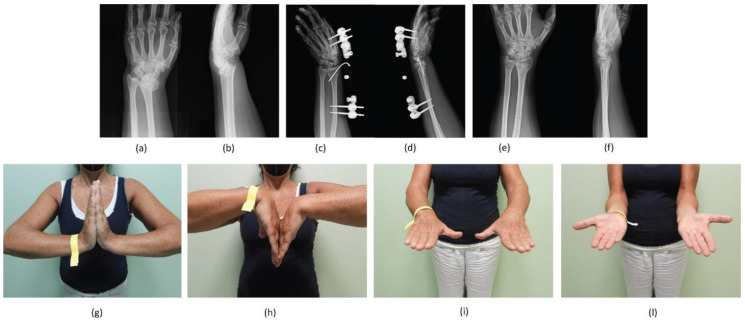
A 58-year-old female patient treated with BEF and an additional KW for an AO 23-C2 DRF. Antero-posterior (AP) and (b) latero-lateral (LL) radiographic images at (a,b) pre-operative period; (c,d) immediate post-operative period; (e,f) at 2-month follow-up. Clinical-functional images showing: (g) extension, (h) flexion, (i) pronation, and (l) supination of the operated wrist at last follow-up.
